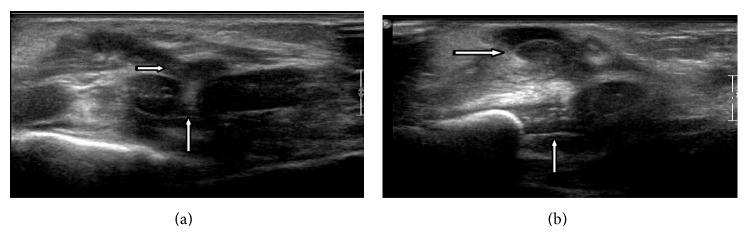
Ultrasonography of a 12-month-old boy presenting chondrosternal arthritis. (a) The route of the joint effusion (stripped arrow), into the chondrosternal joint (arrow). (b) Subcutaneous abscess (stripped arrow) from the chondrosternal joint (arrow).
